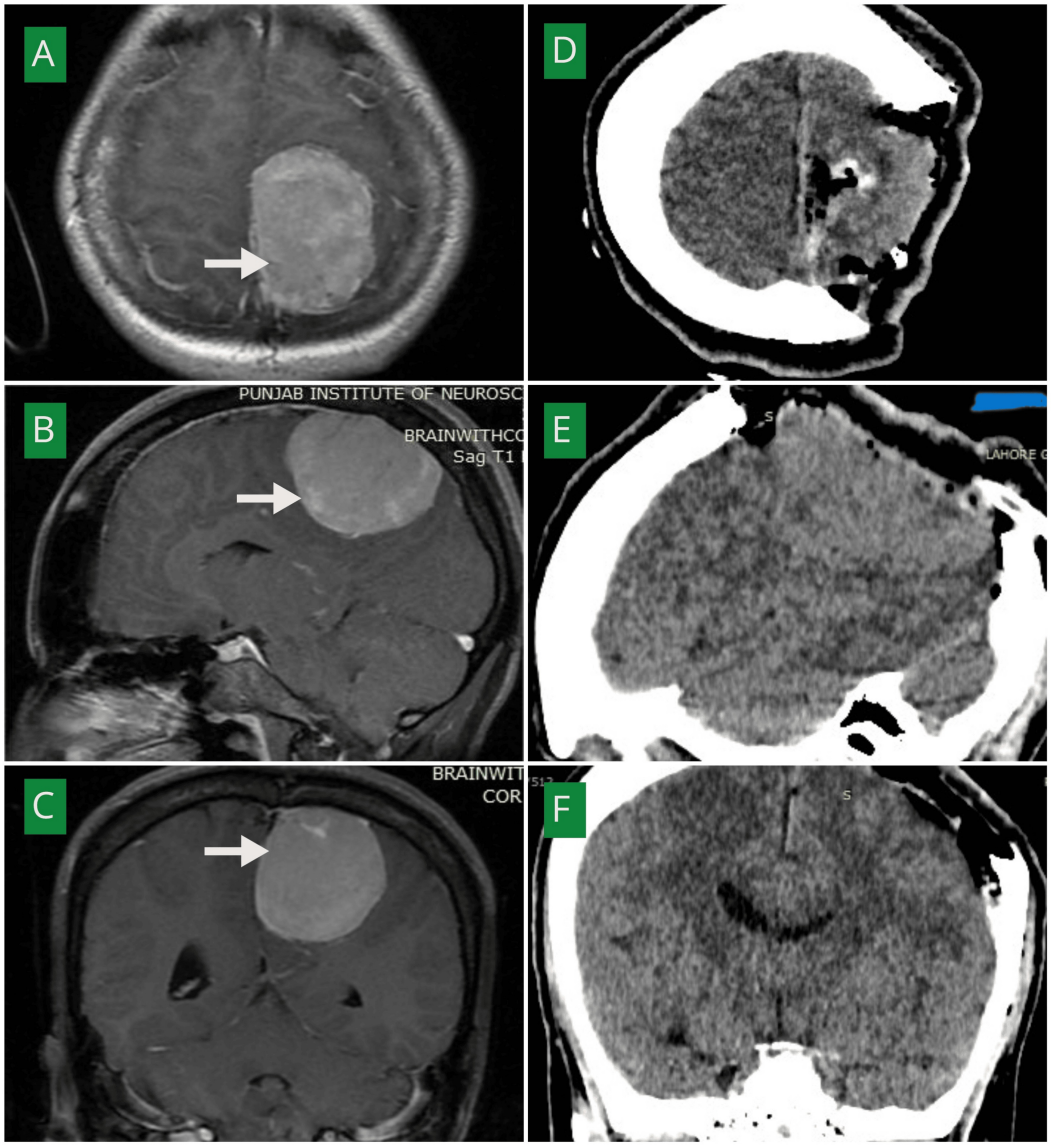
(A–C) Post-contrast MRI brain axial (A), sagittal (B), and coronal (C) views demonstrating a homogeneously enhancing extra-axial mass along the left parafalcine region (white arrows). (D–F) Post-operative non-contrast CT brain axial (D), sagittal (E), and coronal (F) views showing Simpson grade I excision. Postoperative CT scan showing craniectomy performed for tumor excision. The bone was not replaced at the time of surgery due to intraoperative bone involvement and concern for postoperative cerebral edema. Delayed cranioplasty was planned for this patient. MRI: Magnetic resonance imaging, CT: Computed tomography
